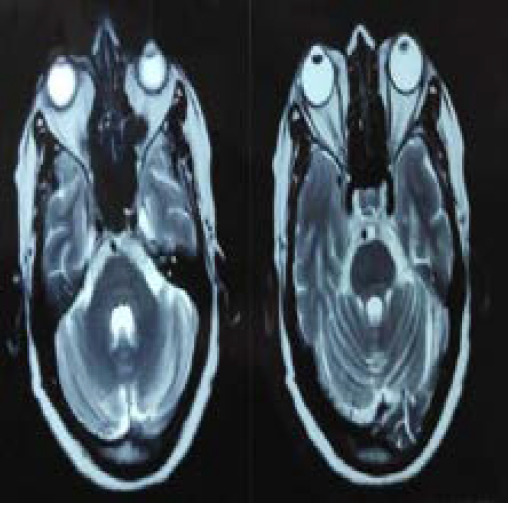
MRI of brain and orbit (Axial) showing increased right optic nerve diameter with altered signal intensity changes in optic nerve and lateral rectus muscle.
